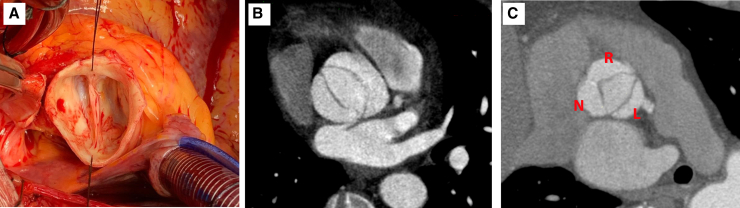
A, Intraoperative view of a 2-sinus BAV with latero-lateral configuration. B, Computed tomography scan view of a 2-sinus BAV, latero-lateral configuration. C, Computed tomography scan view of a raphed BAV with right-non fusion.
